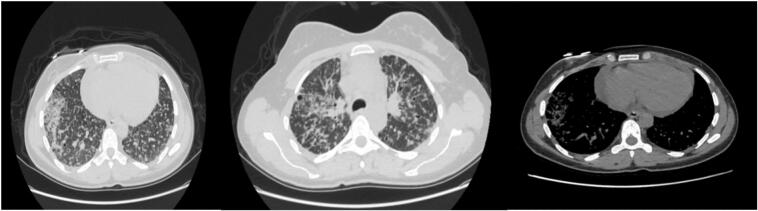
CT chest axial images showing diffuse bilateral interstitial infiltrates, intermixed with ground glass infiltrates and some cystic changes.
